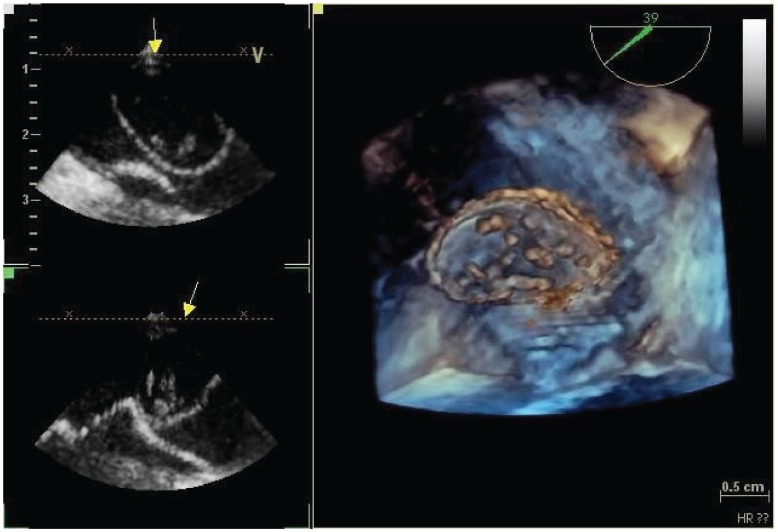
Tridimensional transesophageal echocardiogram of the mass in the left atrium.
